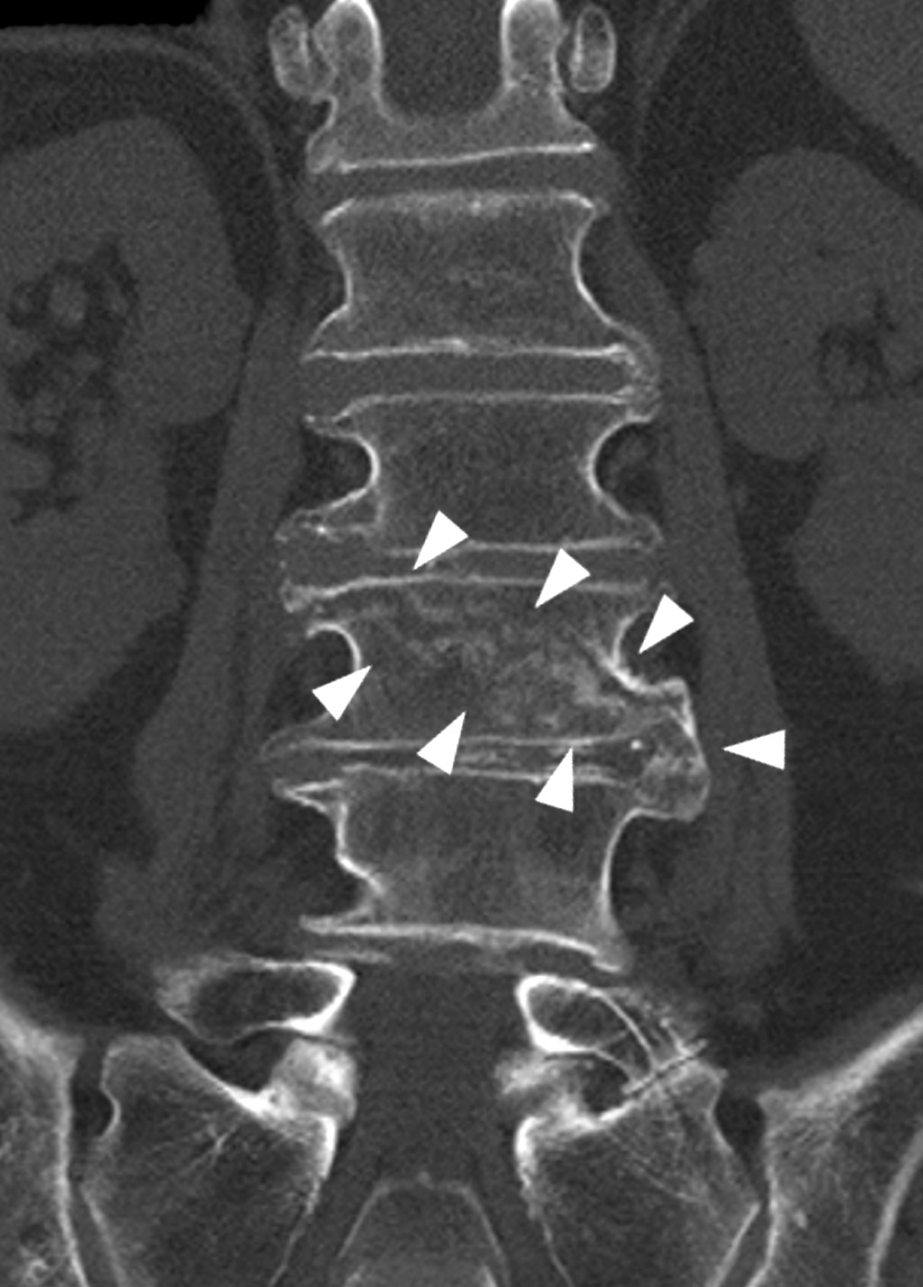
A coronal slice of plain computed tomography performed 2 months after the injury showed callus formation in the fracture gap.
